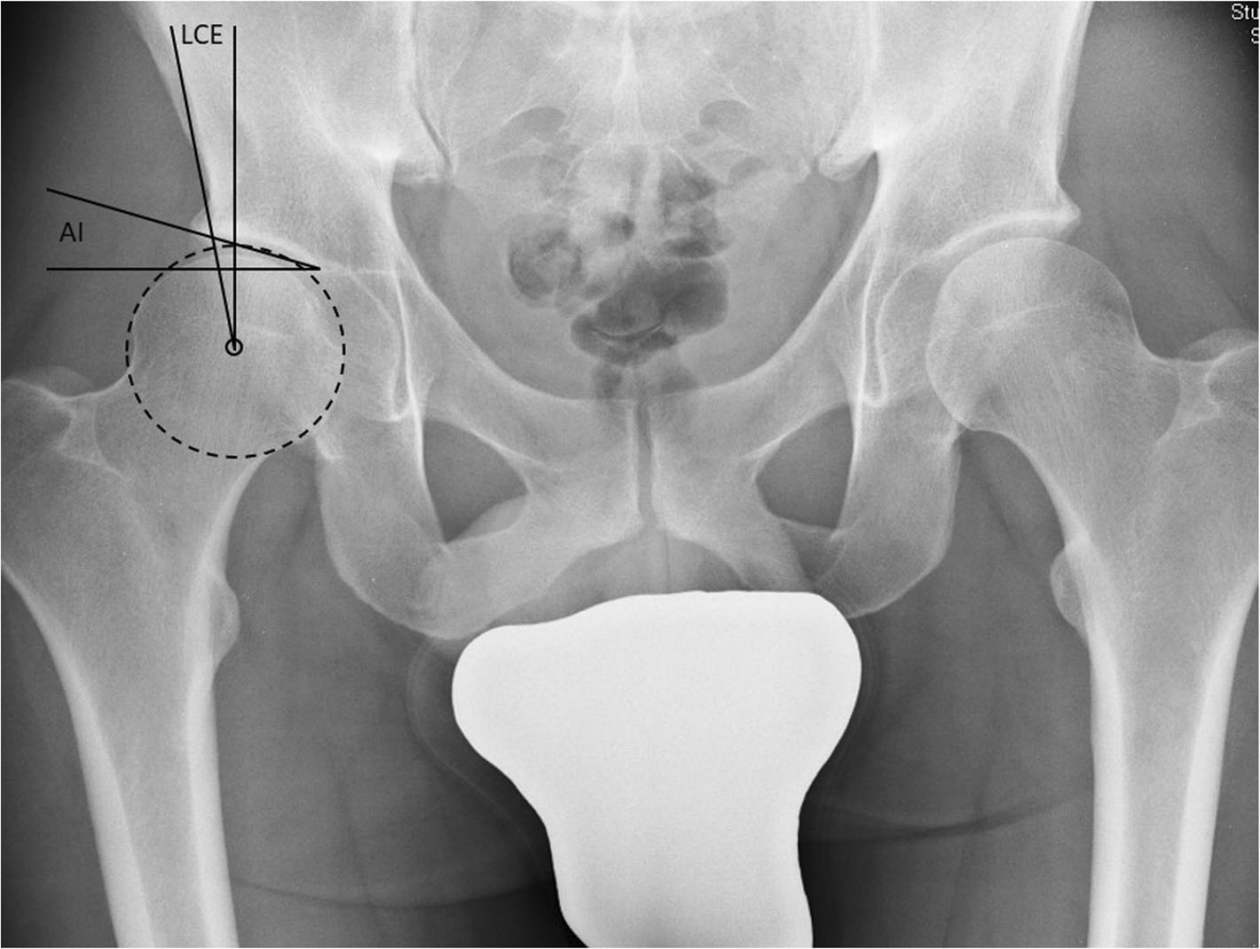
Exemplary radiograph (pelvic view) which shows the measurement of the LCE (Wiberg’s lateral center-edge) and AI (Hilgenreiner’s articular cartilage angle)
